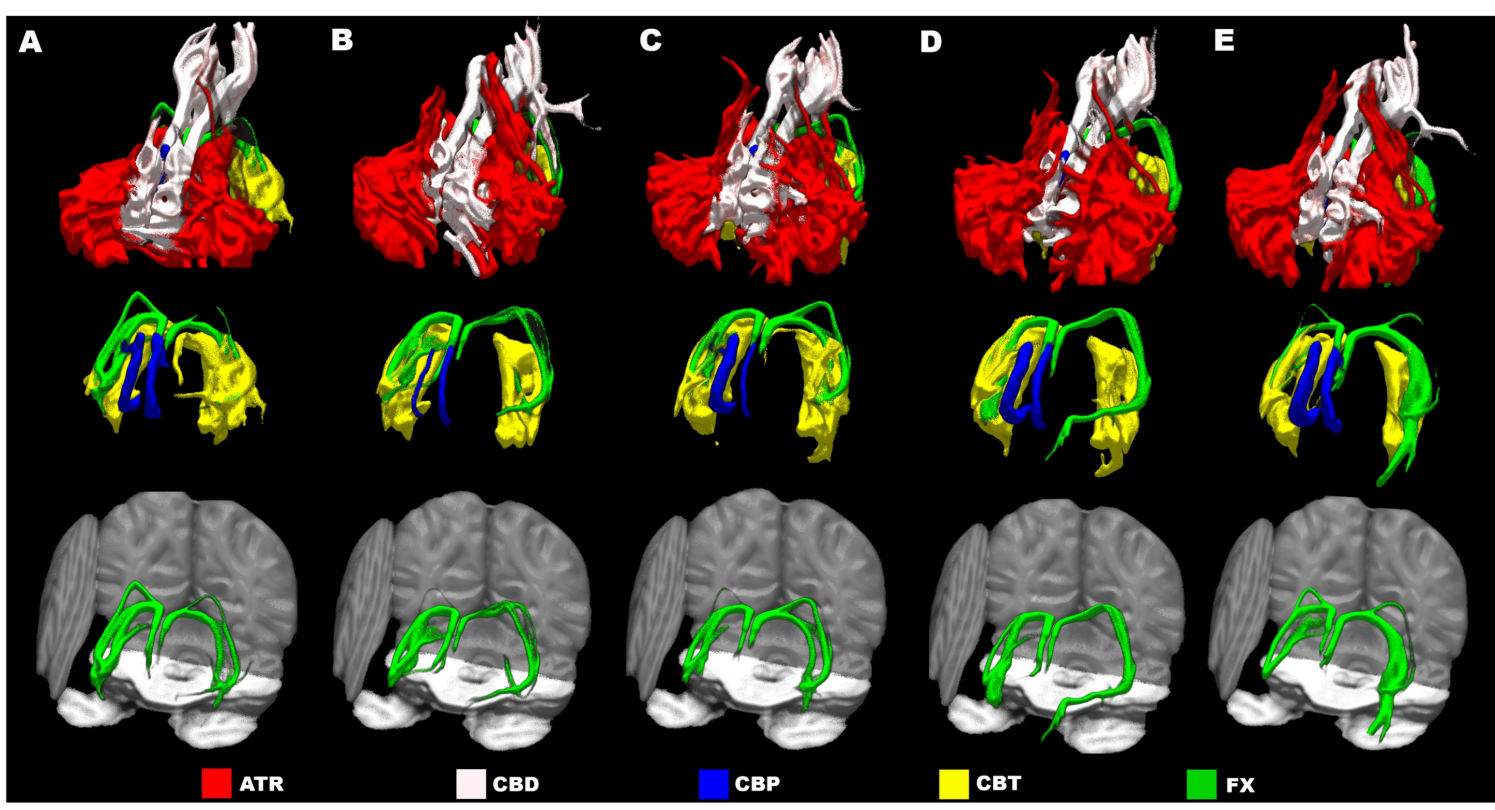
Longitudinal evaluation of tractography related to the Papez circuit Serial tractographic analysis of white matter pathways associated with the Papez circuit using XTRACT on day 1 (A), day 10 (B), day 26 (C), day 55 (D), and day 83 (E). Top row: All tracts associated with the Papez circuit; Middle row: Three tracts, namely the CBP, CPT, and FX, which are partially obscured by ATR and CBD; Bottom row: The FX tract overlaid on individual slices of T1-weighted images. Increased tract visibility, particularly in the FX (red arrowheads), corresponds to longitudinal changes in FA values. Color coding of tracts: Red for ATR, pink for CBD, blue for CBP, yellow for CBT, and green for FX FA: Fractional anisotropy, ATR: Anterior thalamic radiation, CBD: Cingulum subsection dorsal, CBP: Cingulum subsection perigenual, CBT: Cingulum subsection temporal, FX: Fornix
